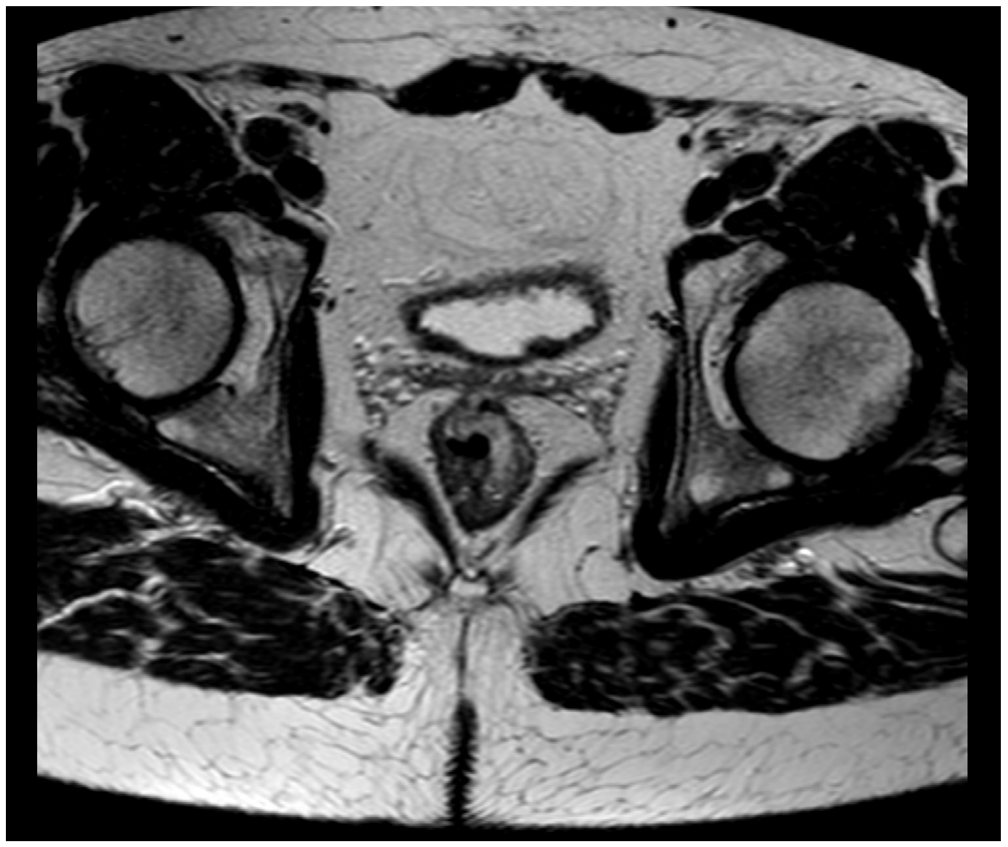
Magnetic resonance imaging scan showing the rectal tumor.
